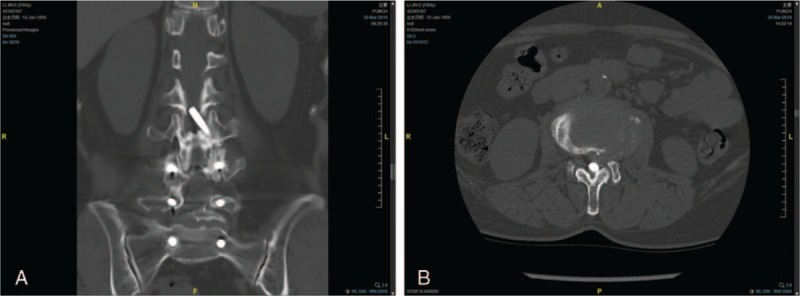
Computed tomography shows (A) coronal reconstruction and (B) an axial section shows intraspinal migration of the left rod.
